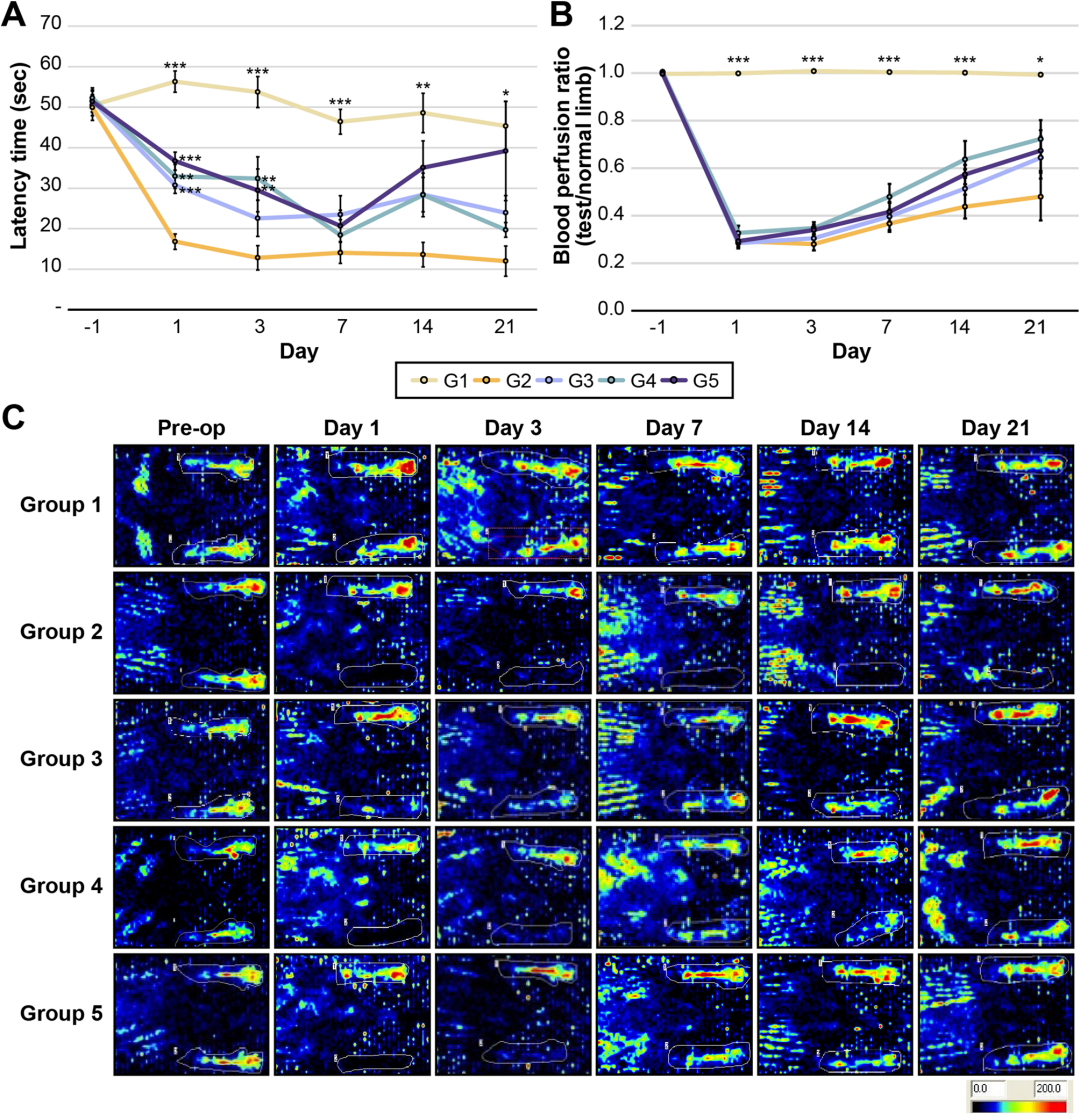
Changes in latency time and blood perfusion ratio. (a) After ischemia induction, the latency time in the E-MSC-treated groups (G3–G5) decreased to less than that in the control group (G2). (b) Compared to G1, the ischemia-induced groups (G2–G5) exhibited significantly low perfusion ratios. While G3–G5 showed a slight trend toward improved perfusion ratios compared to G2, no differences were observed based on E-MSC dosage. However, the perfusion ratios within each group (G2–G5) significantly increased over time, particularly on PODs 14–21 compared with those on POD 1 or 3 (*P* < 0.05). (c) Laser doppler perfusion imaging at different time points. Dark blue indicates low to no blood perfusion, whereas red represents the highest perfusion level. (*, *P* < 0.05, , *P* < 0.01, and *, *P* < 0.001 by the Kruskal–Wallis test).
